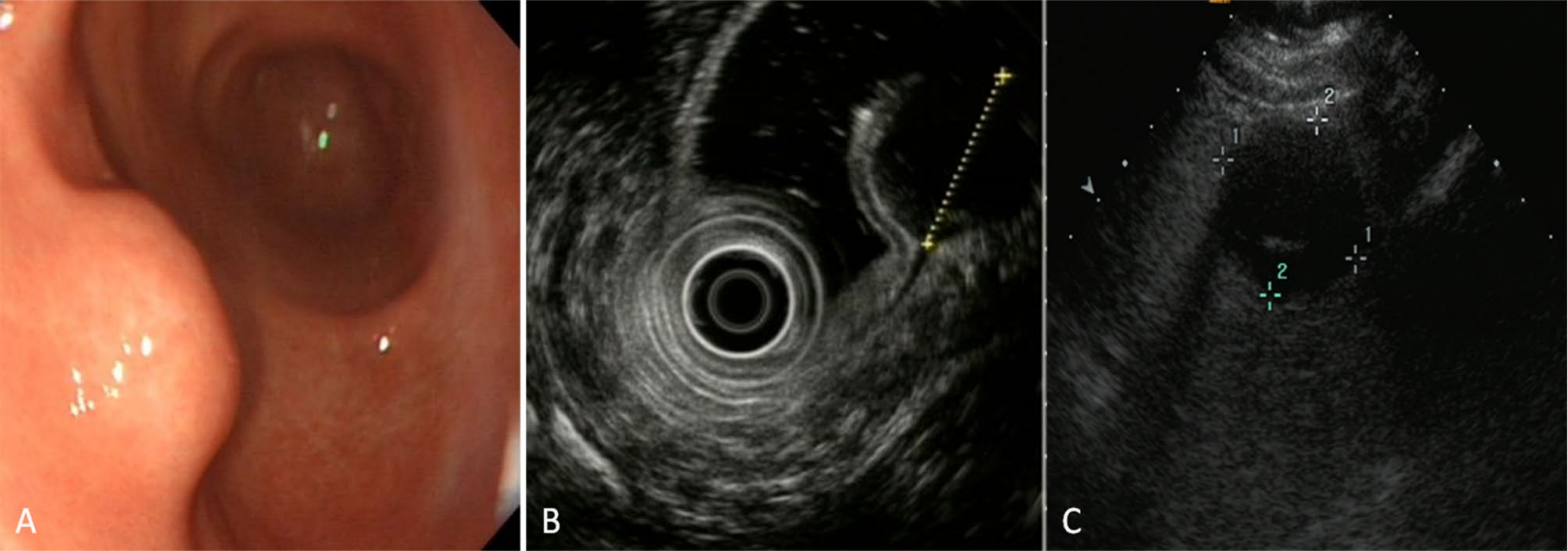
A 53-year-old man with a gastric stromal tumor (GST). (A, B) Endoscopic ultrasonography (EUS) detected a solid, round, and hypoechoic tumor(calipers) in the gastric antrum. (C) Single oral contrast-enhanced ultrasonography (SOCEUS) showed a round, hypoechoic, and heterogeneous mass (calipers) located in the antrum of the stomach
